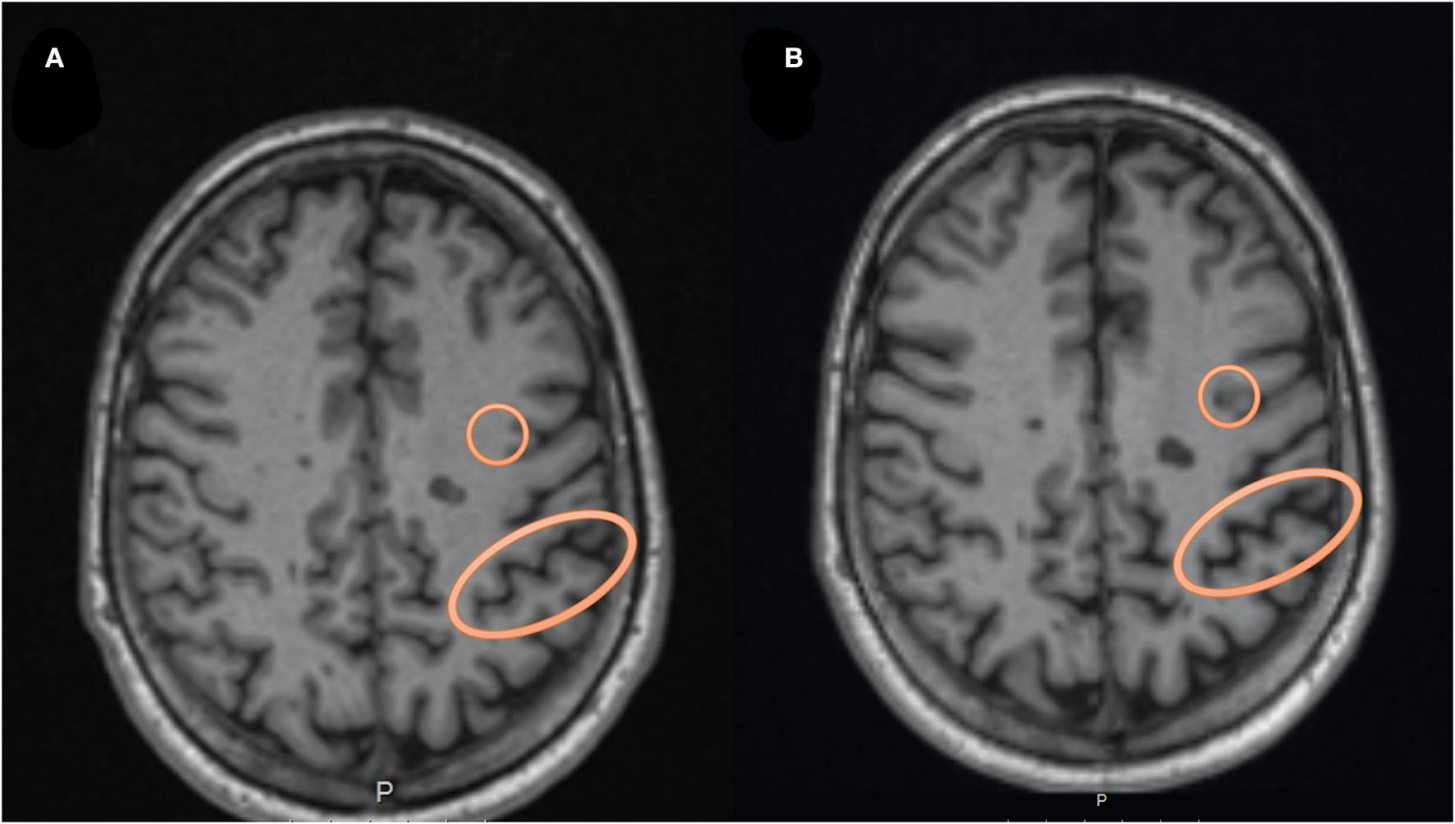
An example of the visual assessment by the neuro-radiologist. In (A) we see an axial T1 MRI at time-point 1, while in (B) we see the MRI at time-point 2, highlighting a circle with an example of a new lesion evolving during the follow-up period. The oval circle is an example of an area showing increased CSF in the sulcus, which was evaluated as representing atrophy between the two time-points.
